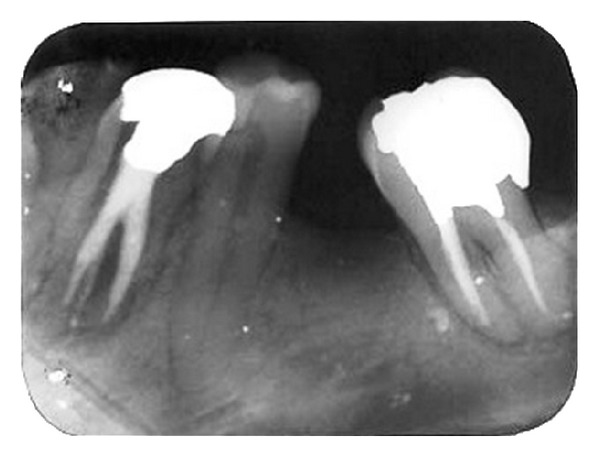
Radiograph revealing complete obturation.
